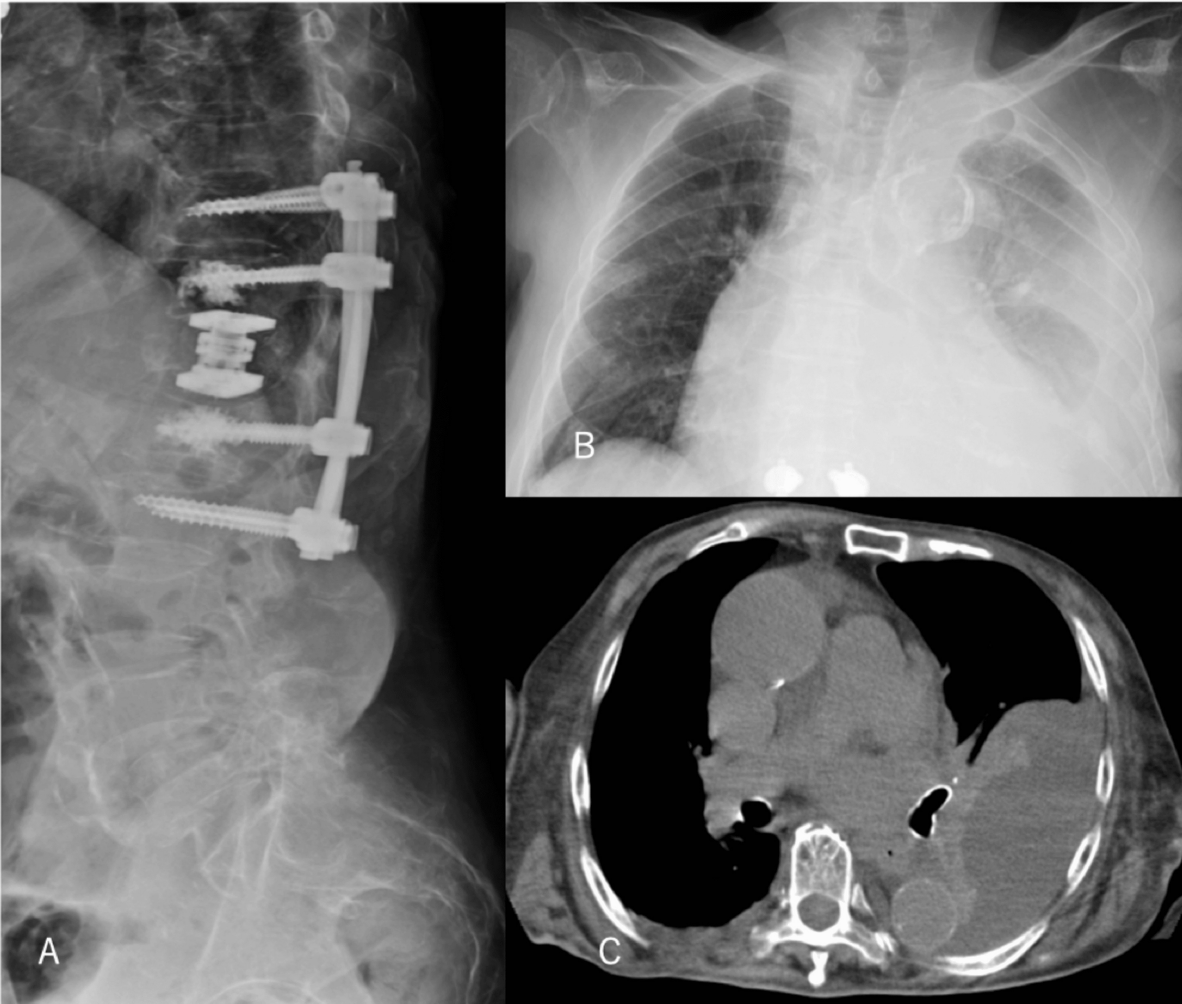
Postoperative Imaging and Development of Pleural Effusion A: Postoperative lateral radiograph demonstrating vertebral body replacement at T12 and posterior instrumentation. B: Chest radiograph on postoperative day 14 showing progressive left-sided pleural effusion. C: Chest CT confirming a large left pleural effusion. Complete resolution of the pleural effusion was confirmed on follow-up chest radiograph after octreotide therapy (not shown).
